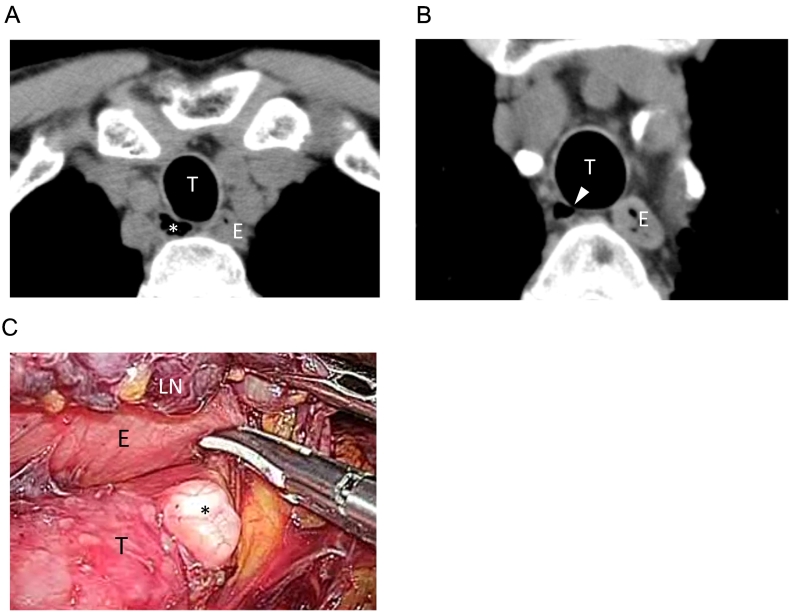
(A) Preoperative CT image of the PTAC in Patient 2 (asterisk). (B) A small connection to the trachea (white arrow head). (C) Intraoperative findings of PTAC in Patient 2 (asterisk). E: esophagus, T: trachea, LN: right para RLN lymph nodes.
